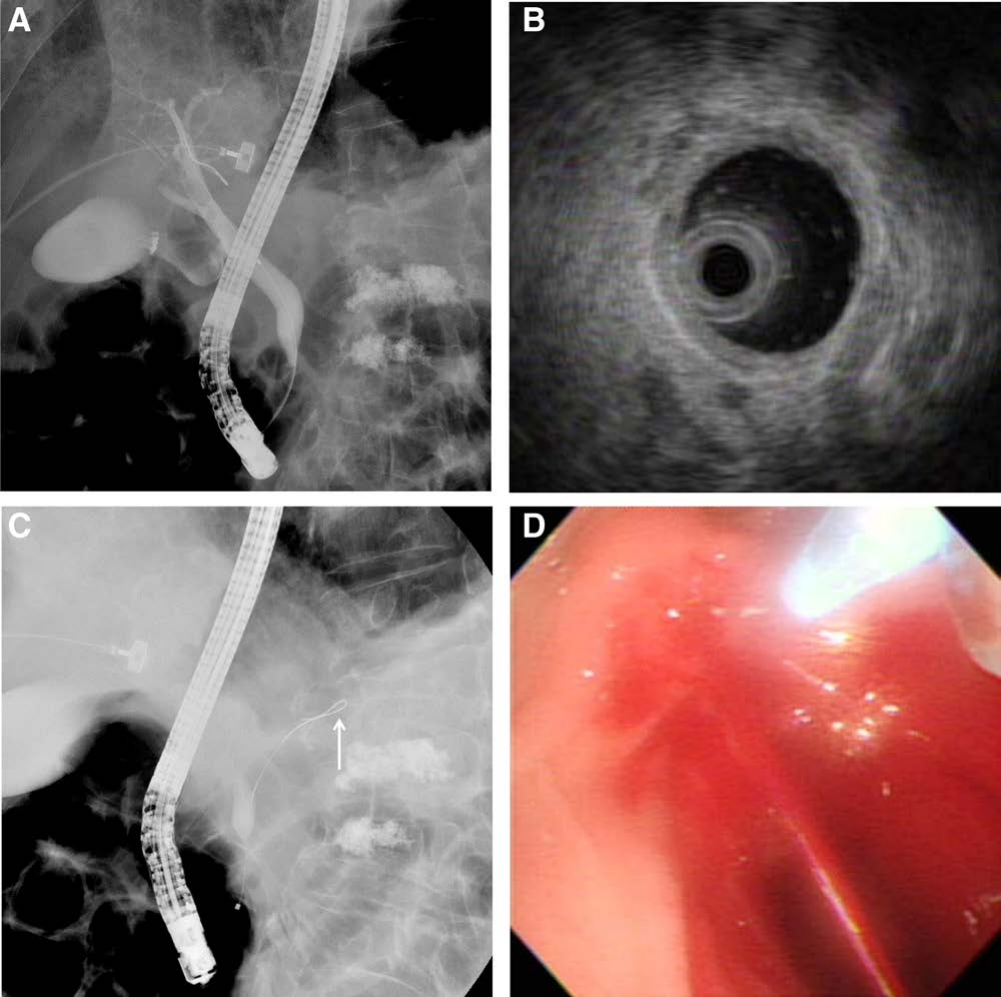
Endoscopic retrograde cholangiopancreatography (ERCP) and intraductal ultrasonography (IDUS) findings. A: ERCP image showing the common bile duct without abnormal filling defects. B: IDUS cross-sectional imaging showing the normal layers of the common bile duct wall; C: The position of the bent tip of a guidewire (arrow) inserted into the main pancreatic duct at the onset of a blood spurt from the major duodenal papilla is observed (IDUS); D: Duodenoscopy showing blood spurting from the major duodenal papilla following a pancreatic duct guidewire placement (IDUS). ERCP = endoscopic retrograde cholangiopancreatography, IDUS = Intraductal ultrasonography.
